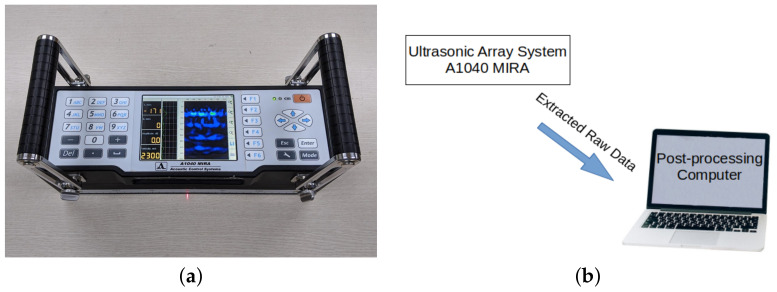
(a) Ultrasonic array system, A1040 MIRA and (b) experiment setup.
